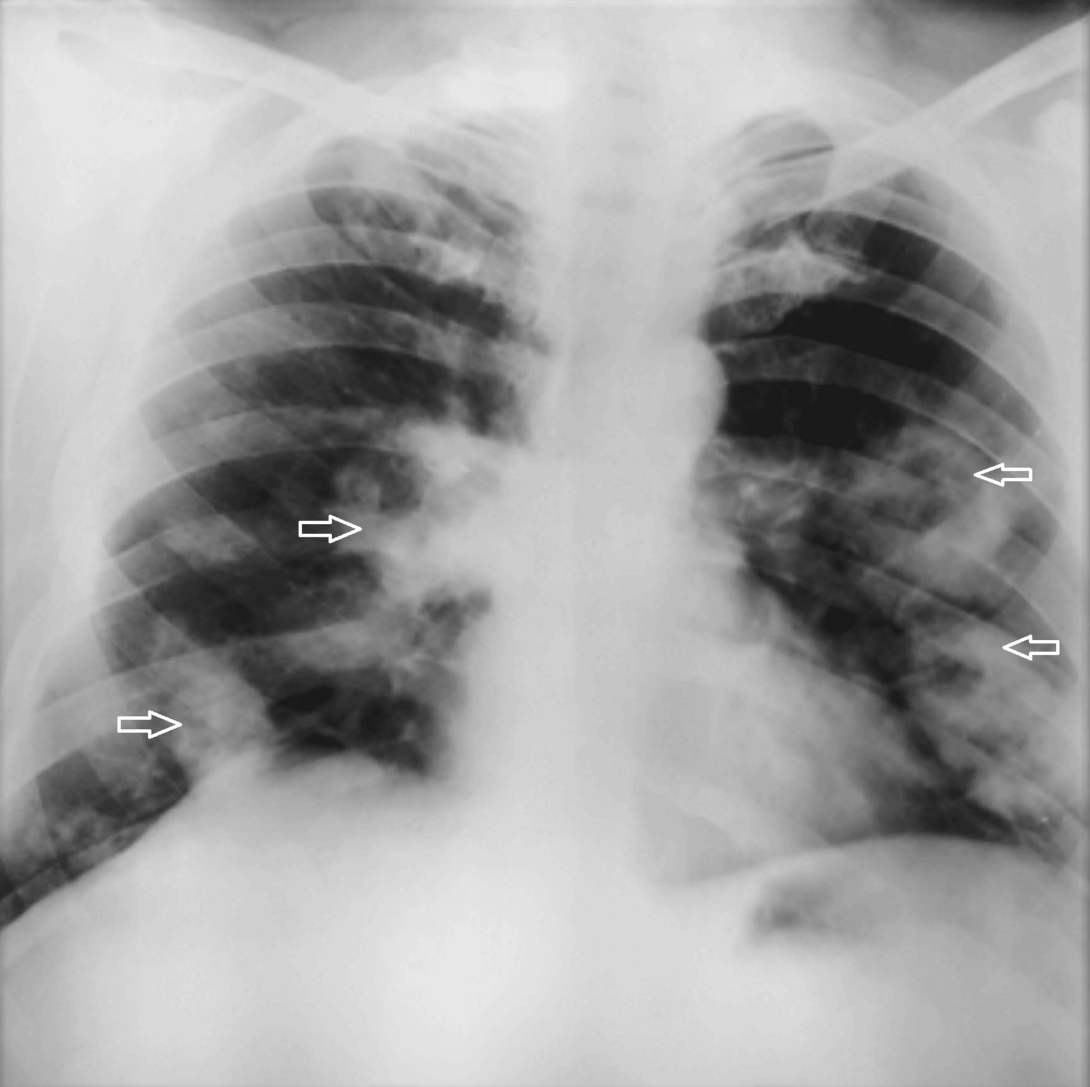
Upright posteroanterior chest radiograph showing multiple bilateral alveolar opacities and areas of consolidation. Arrows indicate opacities with suspected cavitation.
